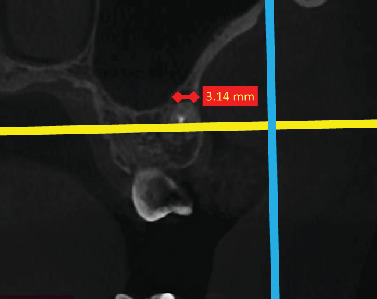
Periapical lesion extension in the coronal view.
